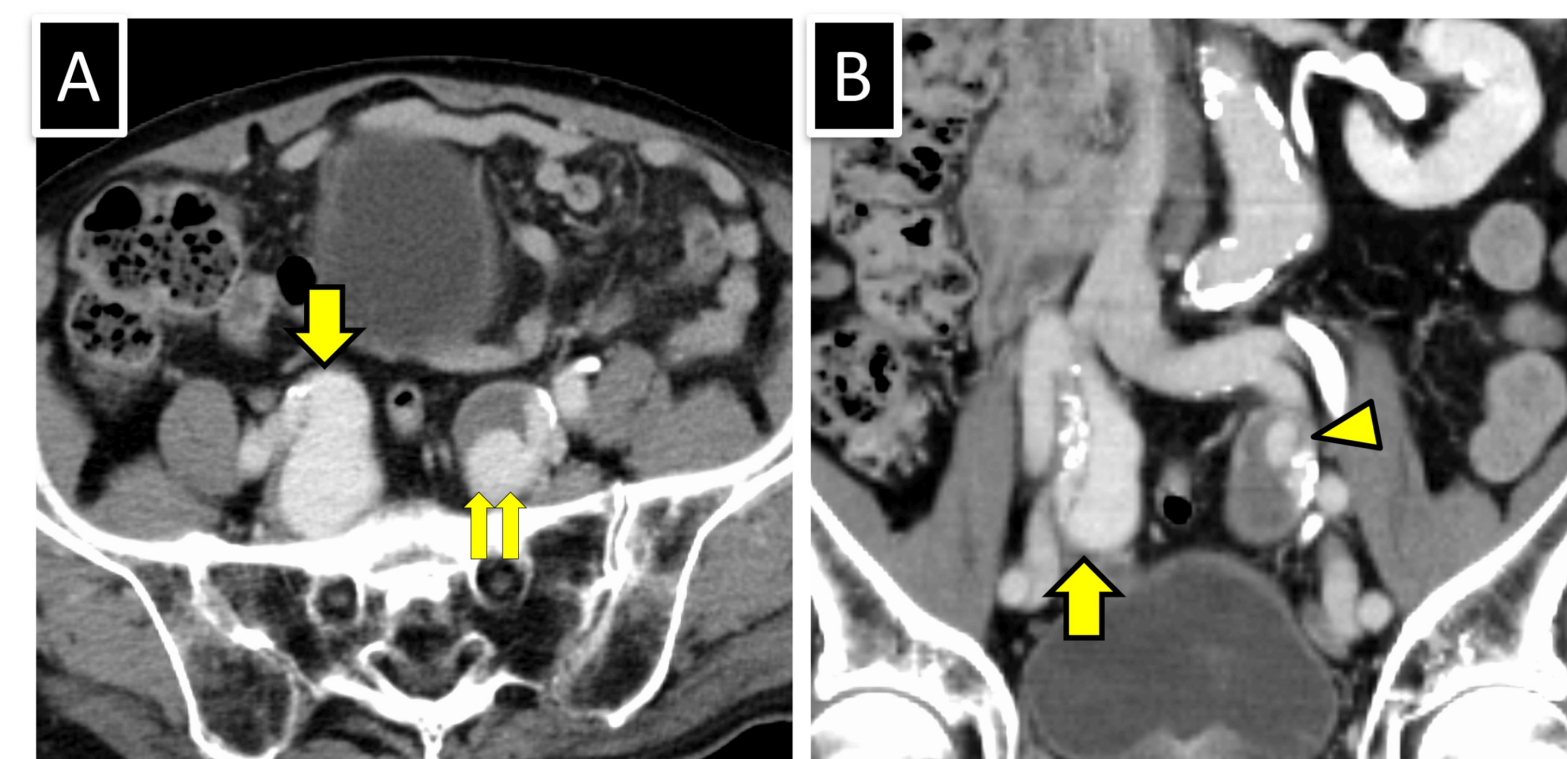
Contrast-enhanced CT 1.5 years post-treatment initiation. (A) In an axial image, a new dissecting aneurysm appeared in the right internal iliac artery (yellow arrow). No significant change was observed in the dissecting aneurysm in the left internal iliac artery, which had partially thrombosed, compared to six months ago. Two patent false lumens are visible: one large (yellow double arrow) and one small lumens. (B) In the coronal image, the right false lumen is not thrombosed (yellow arrow) and true lumen is narrowed due to displacement by intimal calcification. Similarly, the true lumen on the left is narrowed, with a small patent false lumen depicted on the cranial side (yellow arrowhead).
